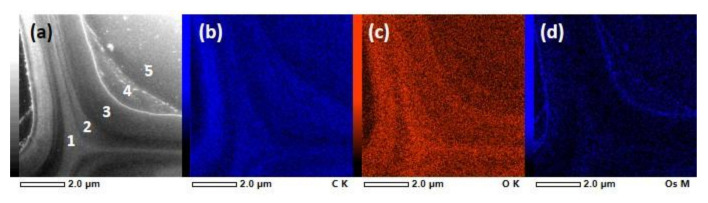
STEM-EDS of the resin-embedded knotwood tracheid’s cell wall structure. (a) STEM image with region numbers corresponding to Table 5. EDS images showing (b) C K α, (c) O K α and (d) Os M α X-ray emission.
